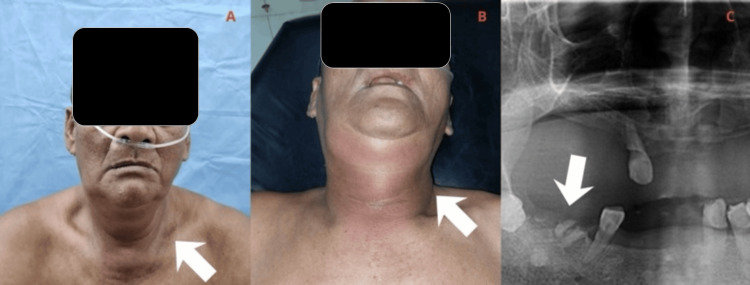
Pre-operative clinical and radiographic presentation A: anterior clinical photograph; B: submental view of the 72-year-old male demonstrating rapidly progressive, diffuse swelling and erythema originating from the right submandibular space and extending across the midline toward the anterior chest wall (arrow); C: cropped panoramic radiograph oriented to the right mandible, revealing severe dental caries and radicular involvement of the right posterior teeth (arrow), identifying the odontogenic source of the phlegmon. Written informed consent to include this image in an open-access article was obtained from the patient.
